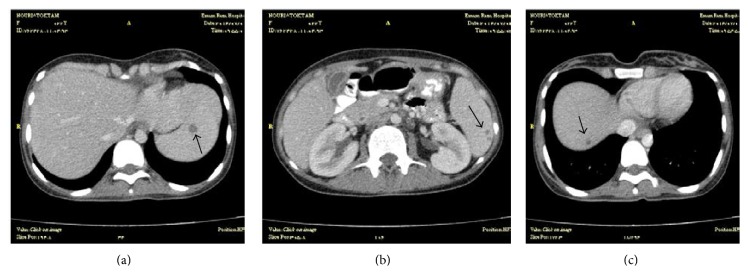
Computed tomography of the abdomen and pelvis with intravenous and oral contrast reveals multiple hypodense lesions in spleen ((a) and (b)) and liver (c).
